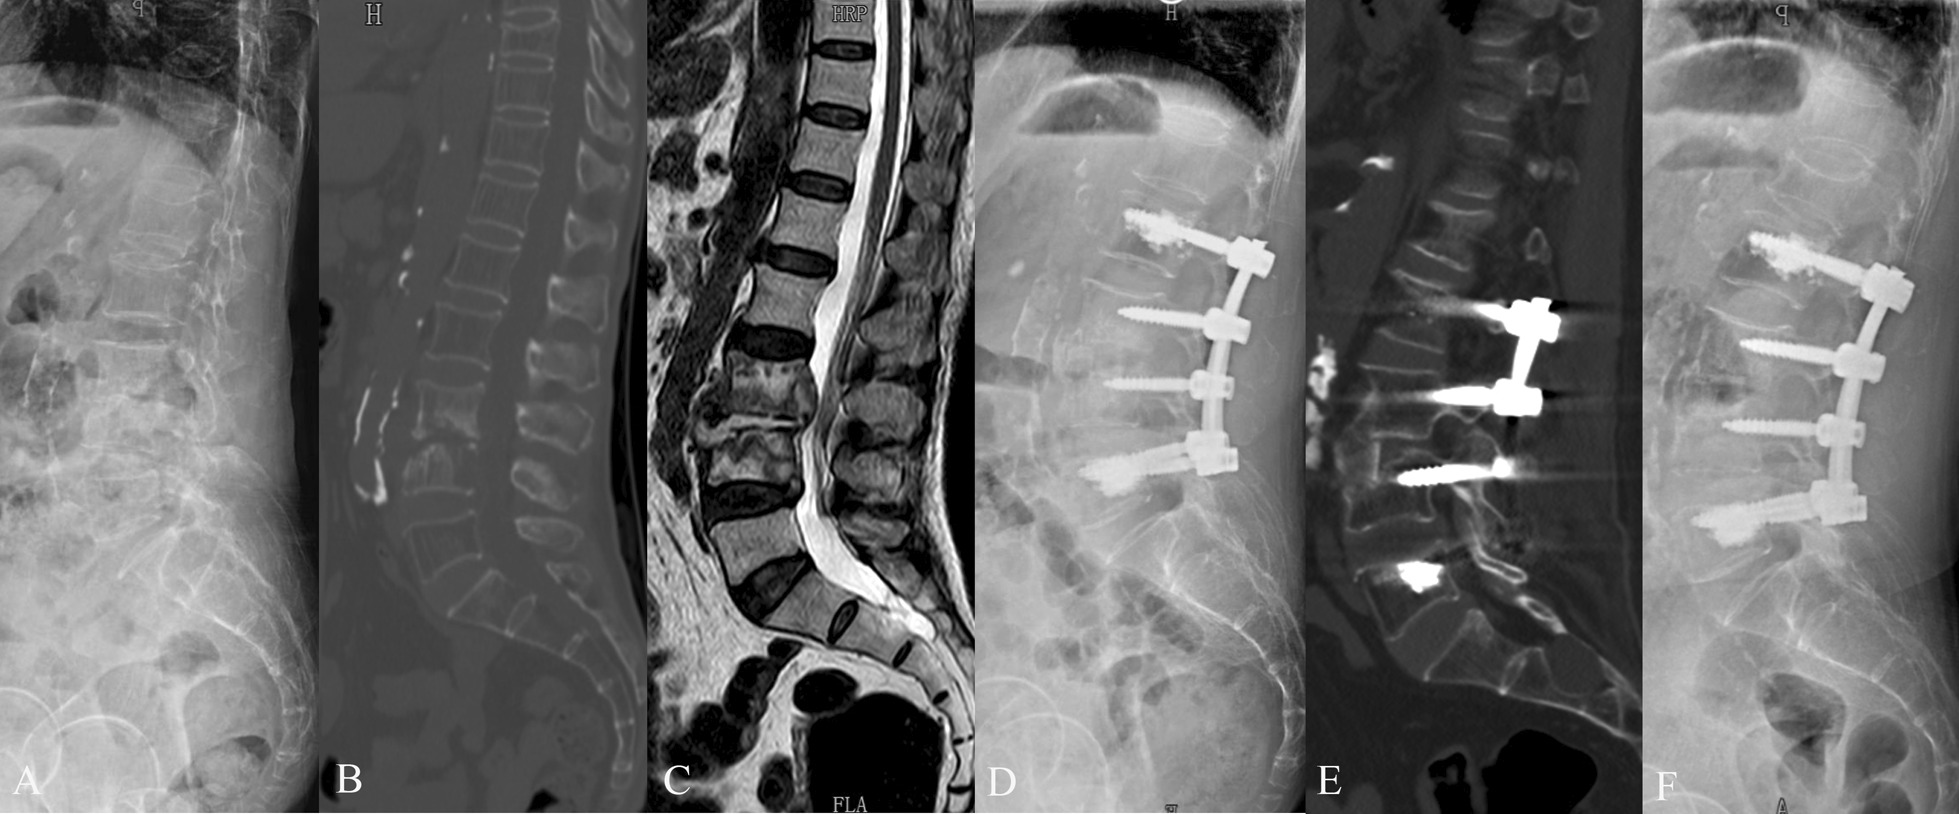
A 72-year-old female with L3-4 tuberculosis and severe osteoporosis. The patient underwent one stage posterior debridement, bone grafting, and fixation with cement-augmented pedicle screws. A–C Preoperative lateral X-ray, sagittal CT scan, and sagittal MRI scan images demonstrated bone destruction and intraspinal abscess. D Postoperative lateral X-ray image. E Sagittal CT scan at 8-month follow-up. F X-ray image at 25-month follow-up
